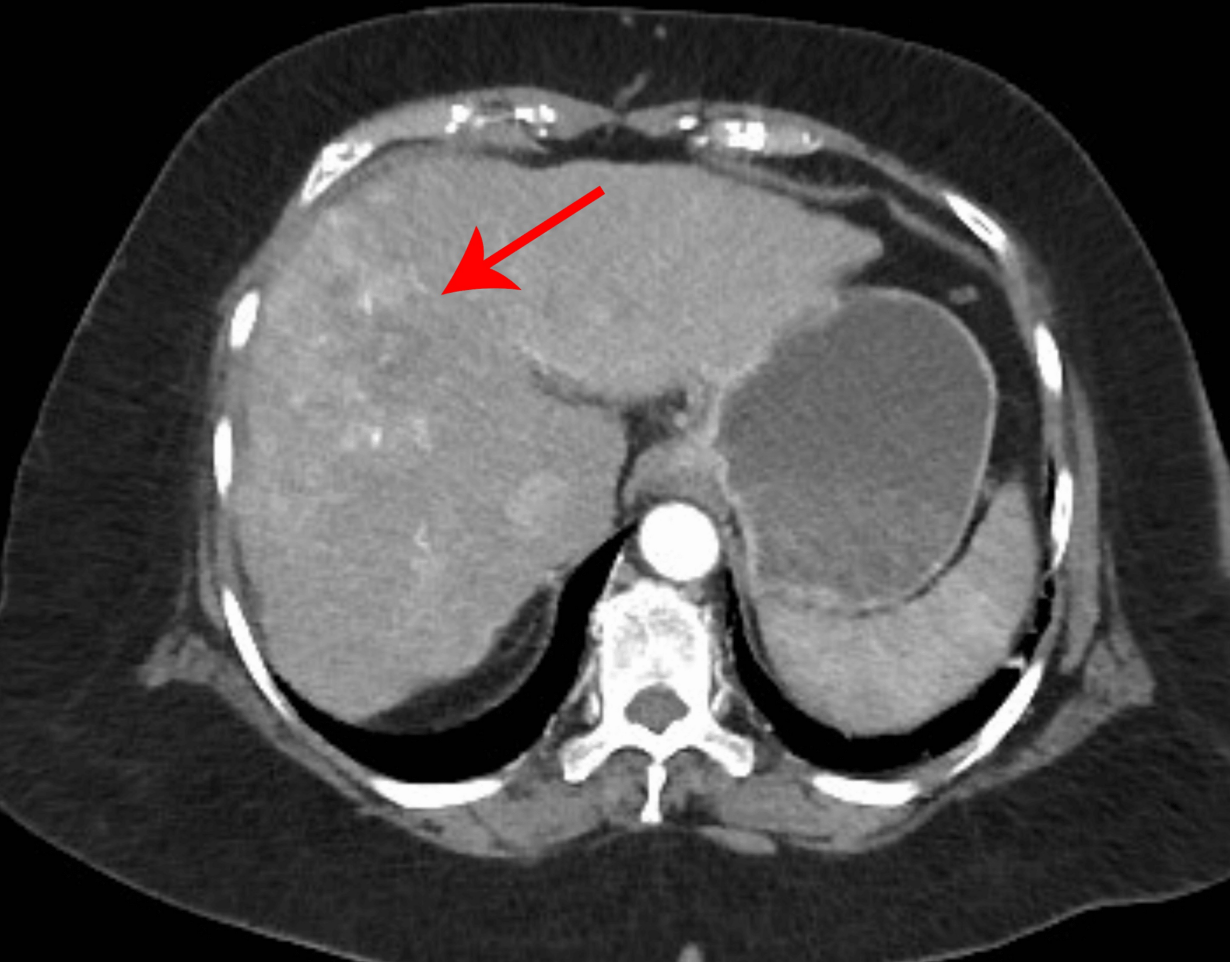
Arterial phase of triphasic CT scan of liver The image shows a contrast-enhanced CT scan of the liver demonstrating multiple variably sized lesions with arterial phase enhancement. These lesions appear brighter than the surrounding normal liver parenchyma. The red arrow highlights one of the hyperenhancing lesions, a typical imaging feature of hepatocellular carcinoma (HCC).
